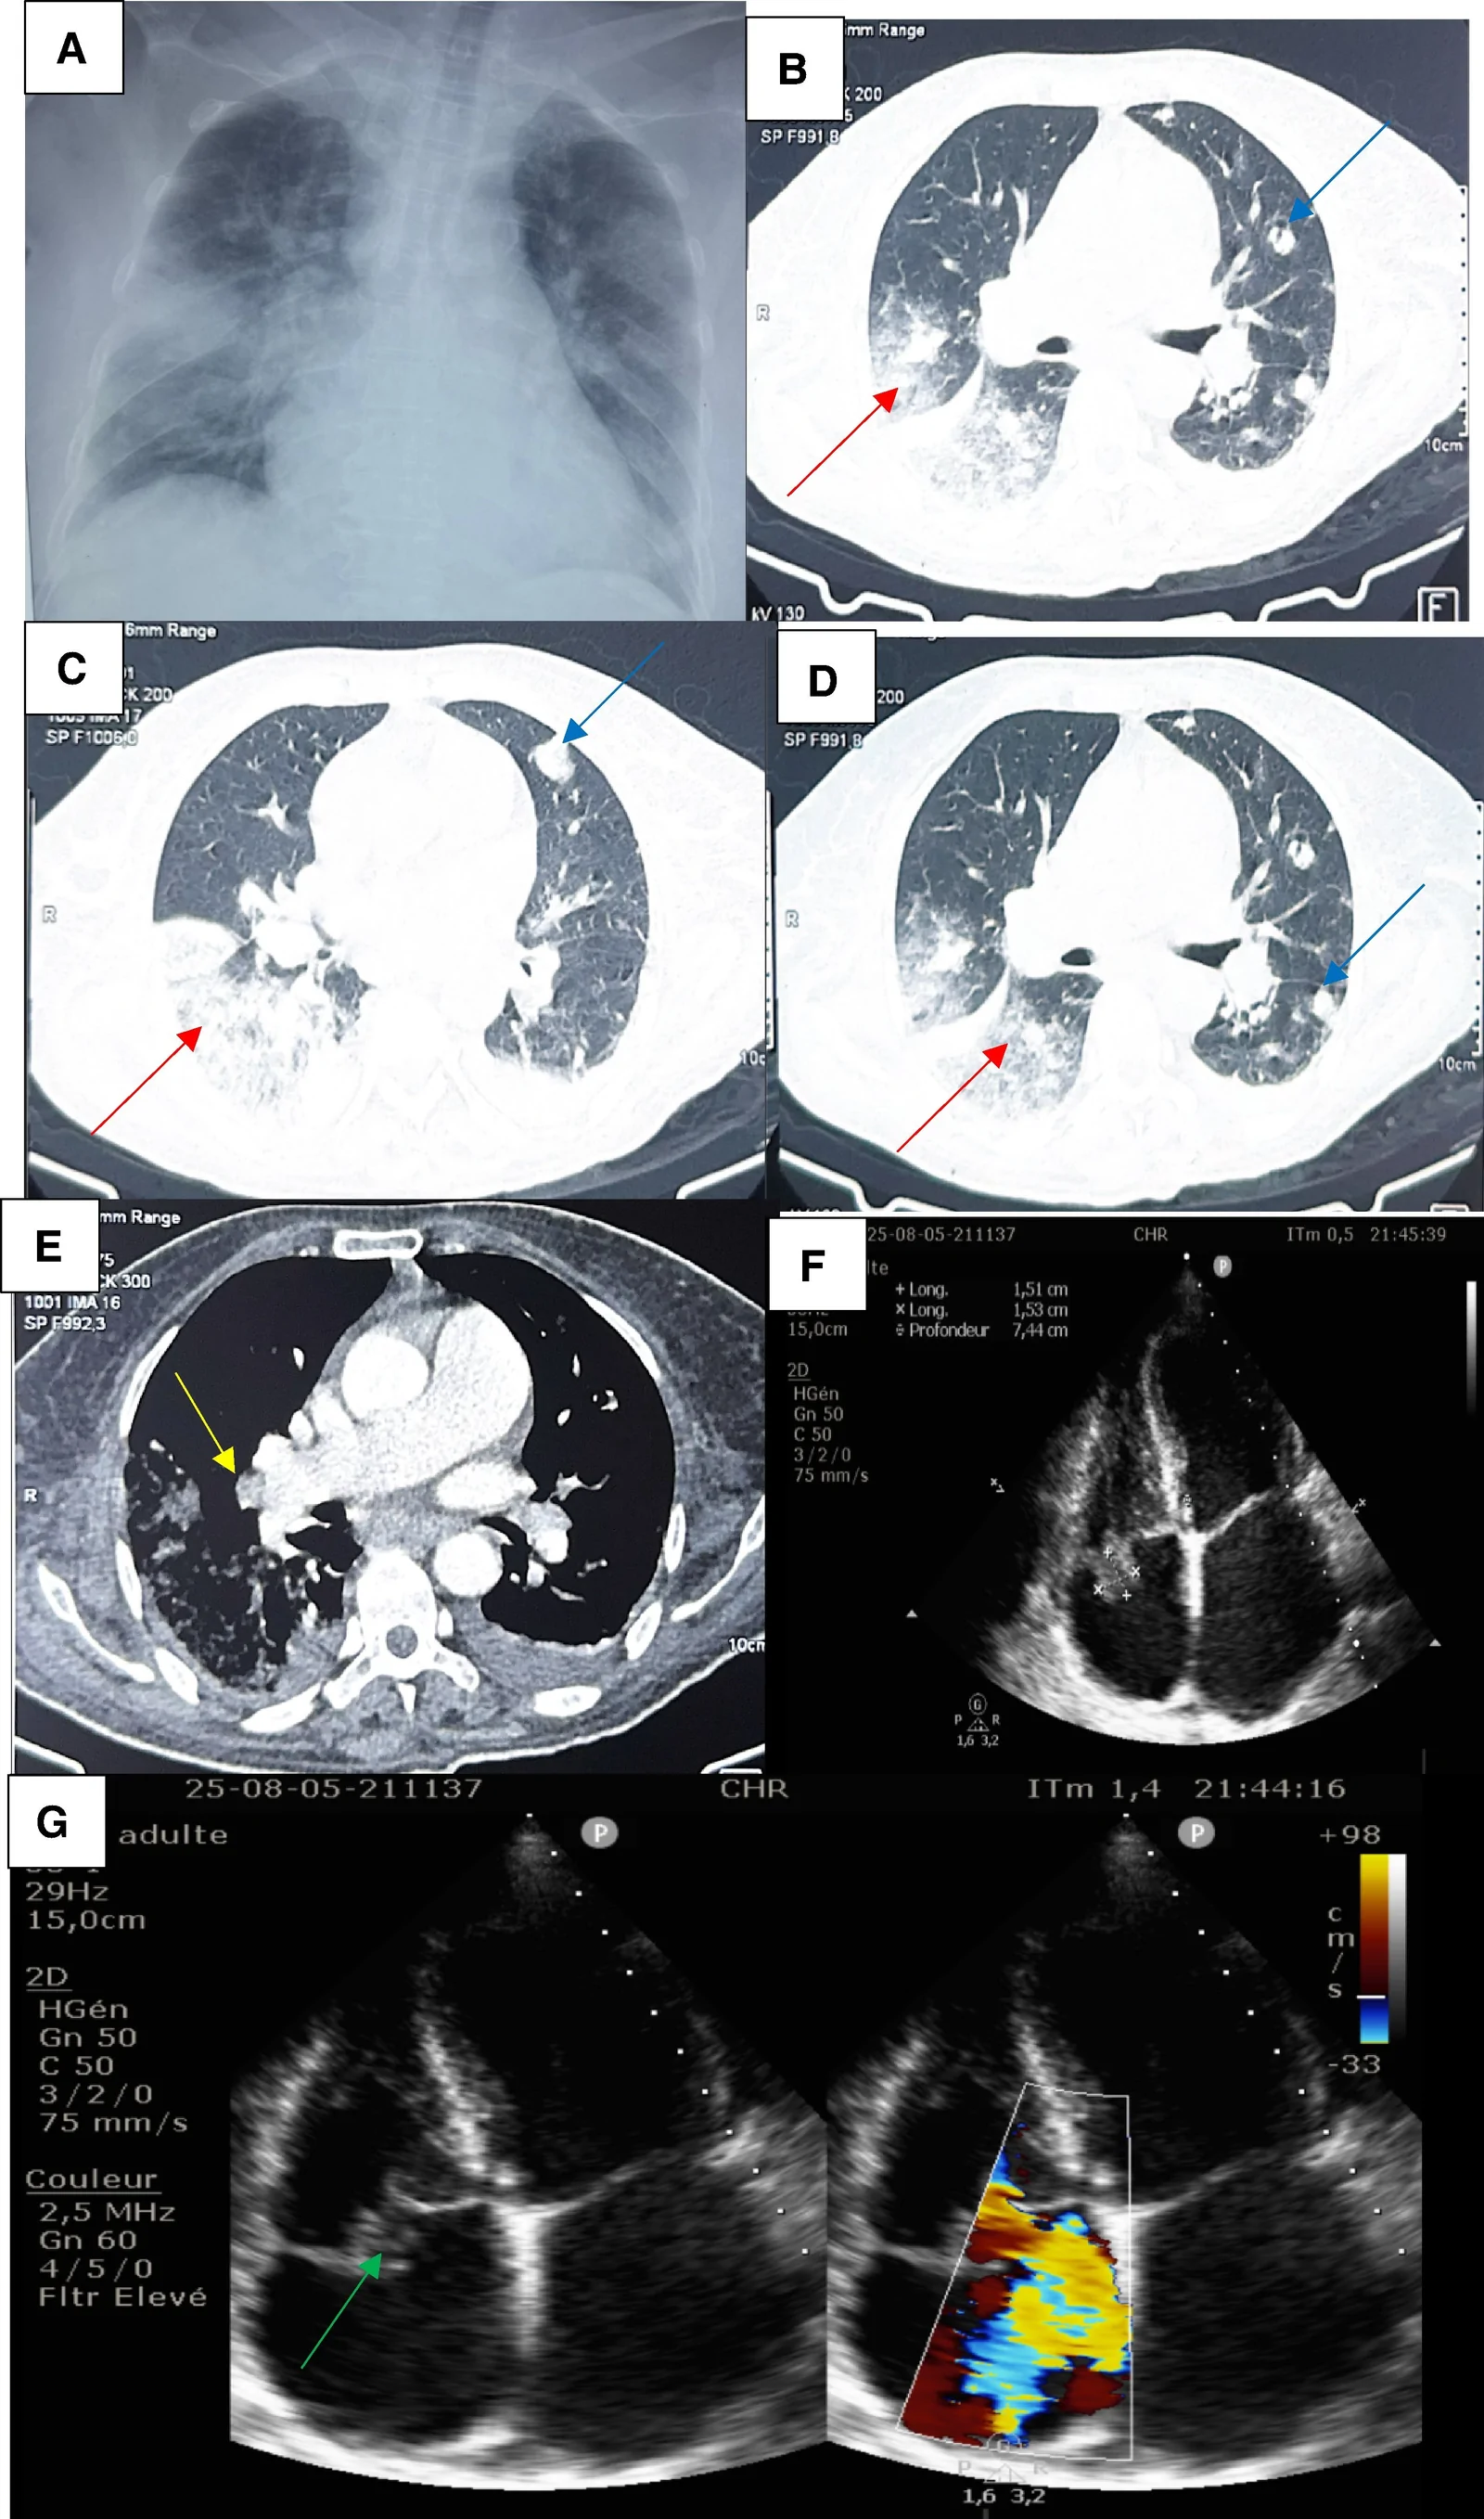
(*A*) Chest X-ray showed cardiomegaly, straightening of the pulmonary arch, and nodular pulmonary infiltrates in both lungs with a localized right pulmonary opacity. (*B–E*) The chest CT scan showed multiple consolidative areas with ground-glass opacities (red arrow), multiple bilateral pulmonary nodules (blue arrow)—most of which show a feeding vessel sign—a segmental septic pulmonary embolism (yellow arrow), and bilateral pleural effusion. (*F, G*) Transthoracic echocardiography revealed a mobile structure measuring 15 × 15 mm, adherent to the posterior leaflet of the tricuspid valve, causing leaflet prolapse (green arrow) and resulting in eccentric moderate tricuspid regurgitation.
